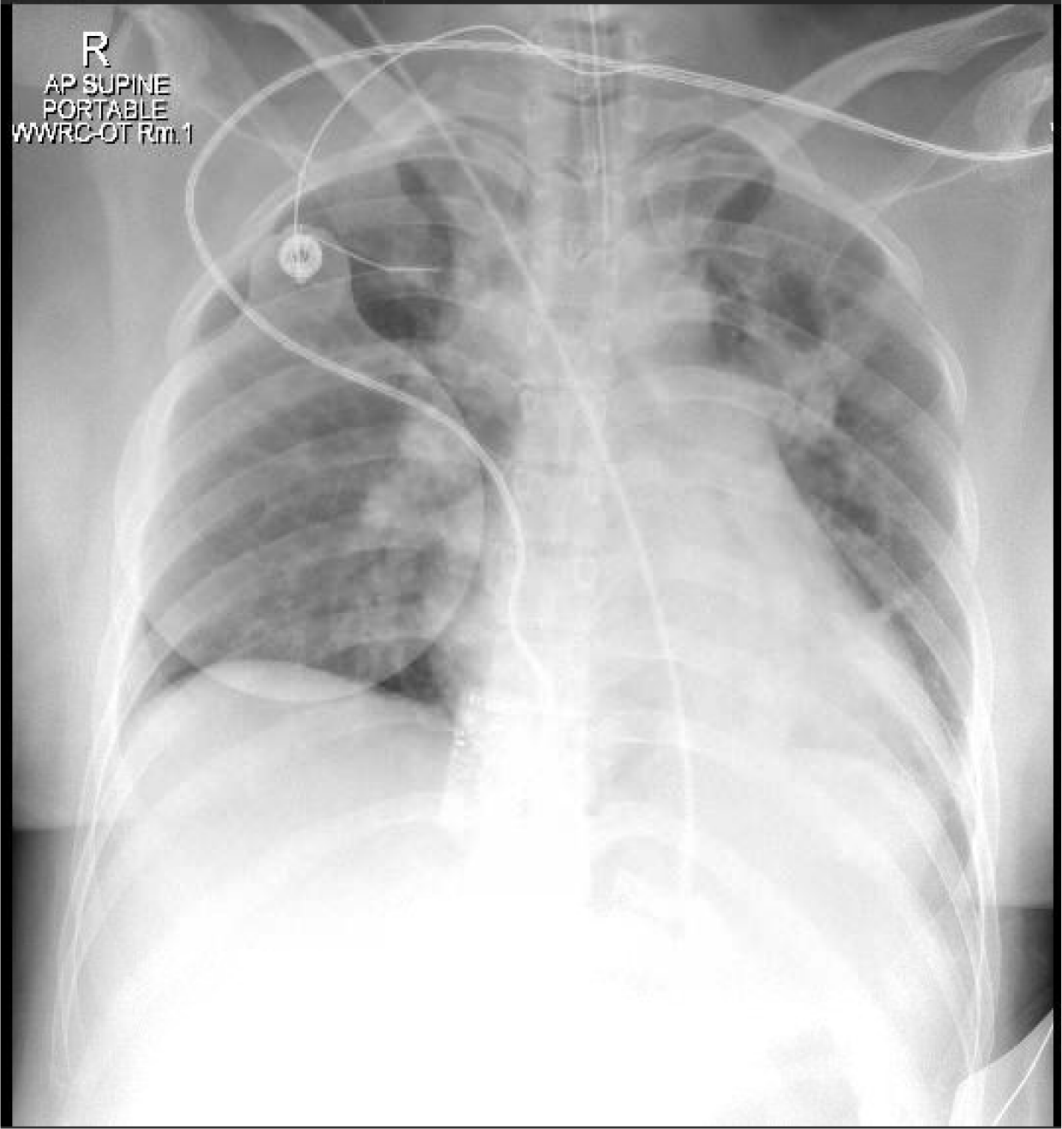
Patient’s chest X-ray showing pulmonary congestion post-cardiac arrest.
